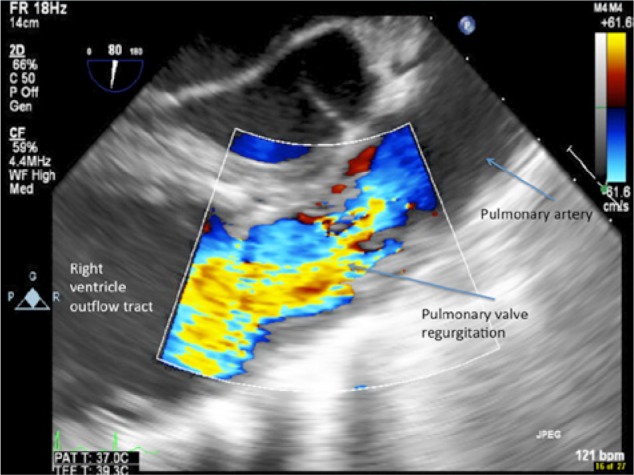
Transesophageal echocardiogram pulmonary outflow view showing severe pulmonary insufficiency.
